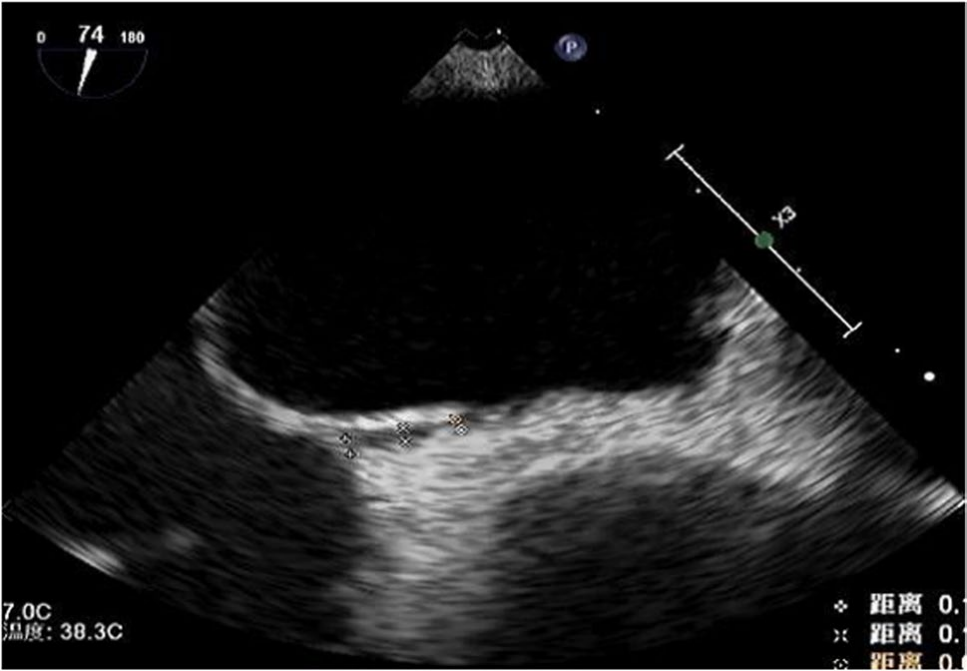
Transesophageal echocardiography shows the right atrial separation of the patent foramen ovale detected at the fossa ovalis in the middle of the interatrial septum
